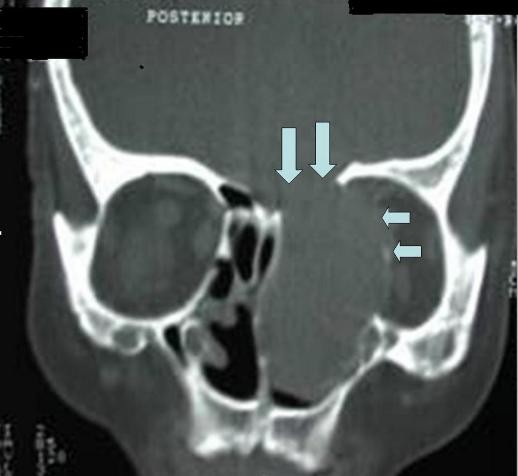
Coronal computed tomography (CT) scan of our patient with cholesteatoma inside the concha bullosa, erosion of lamina papyracea (small arrows) and ethmoid roof (large arrows).
